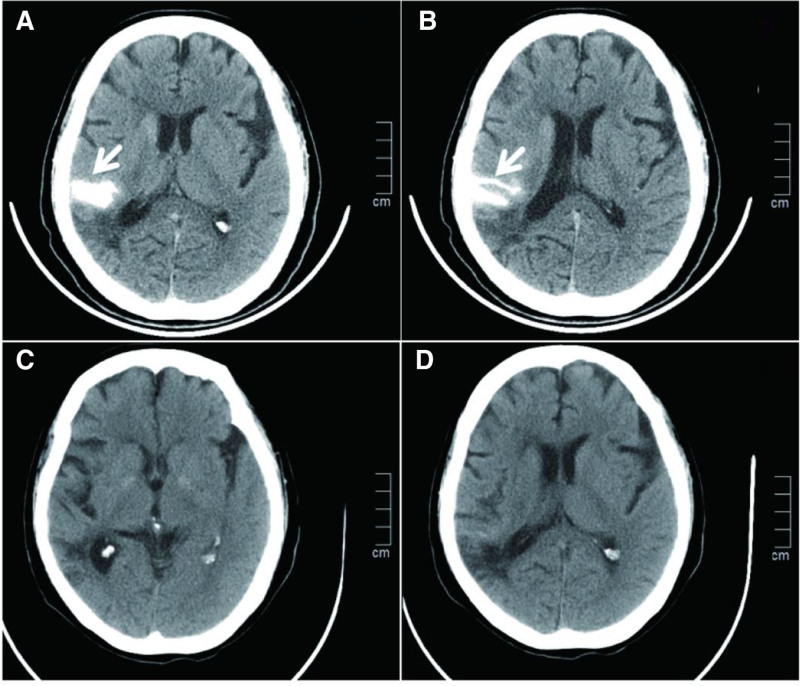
Postoperative cranial CT scans. (A, B) Scans show minimal subarachnoid hemorrhage; (C, D) Subsequent scan reveals complete resolution. CT = computed tomography.
